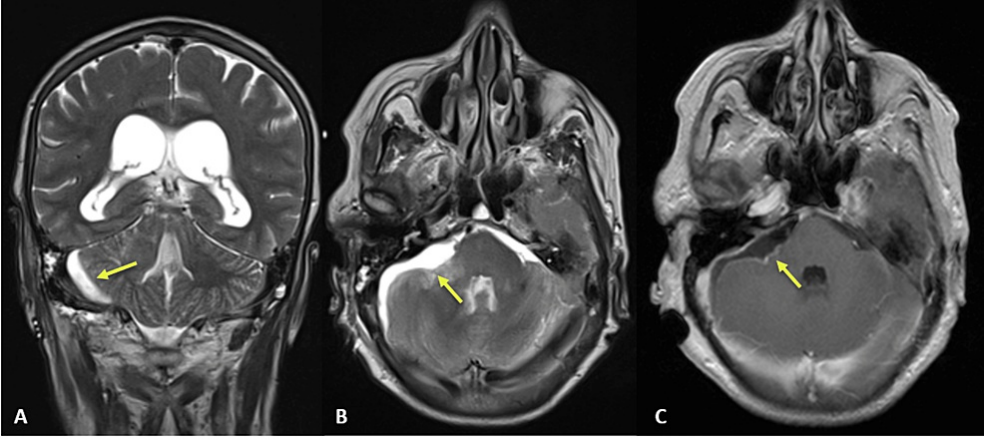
Post-operative cranial MRI, showing gross removal of the lesion. (A) Coronal T2; (B) axial T2; (C) gadolinium-enhanced axial T1. Yellow arrow indicating surgical site.
